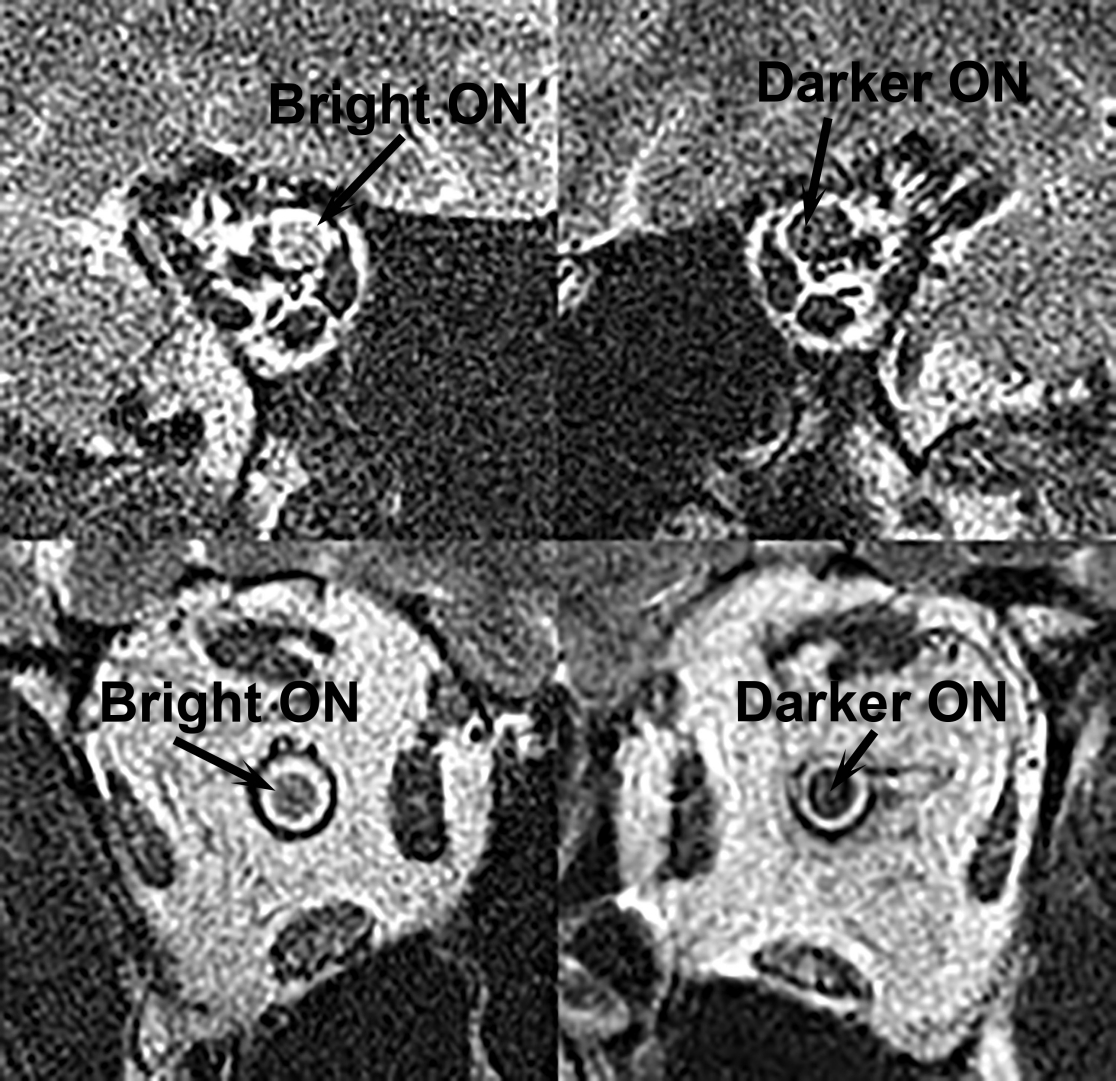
Subacute bright signal in the optic nerve (ON) in NA-AION. Quasi-coronal MRI of the right and left orbits of a 64-year-old woman imaged 42 days following acute onset of NA-AION in the right eye.
